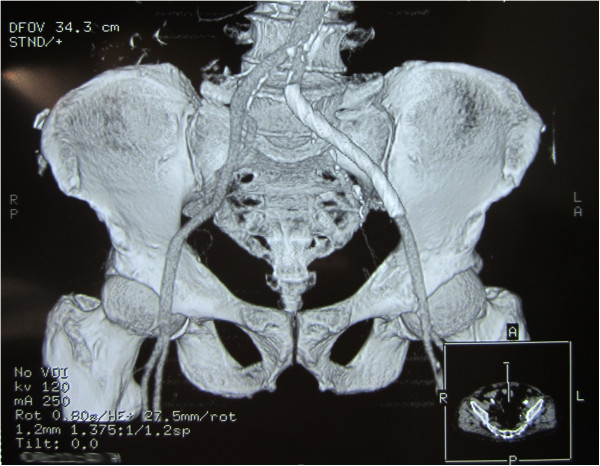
Imaging of computed tomographic angiography after 30 days of discharge. Contrast-enhanced computed tomographic angiography after 30 days of the treatment revealed a well-expanded stent and total occlusion of pseudoaneurysm.
